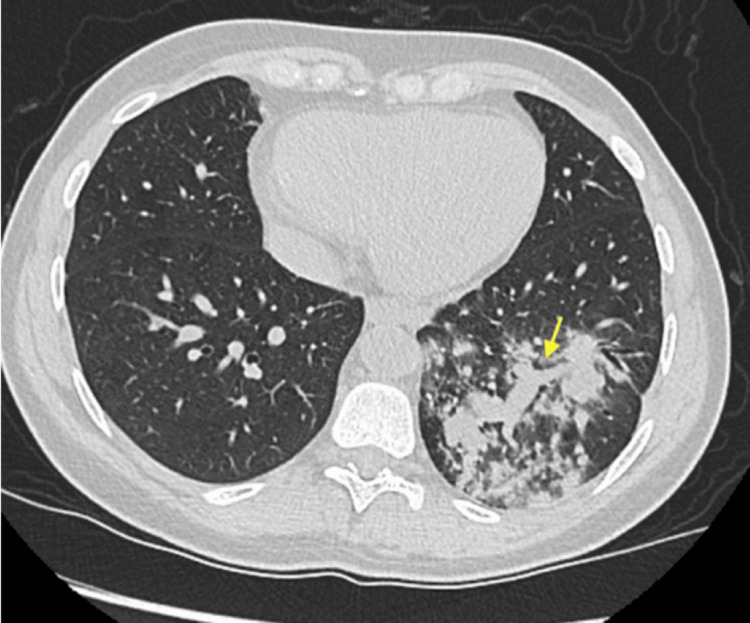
Spiral chest computed tomography (CT) demonstrated a cavitating lesion within an area of dense left lower lobe consolidation (yellow arrow).
